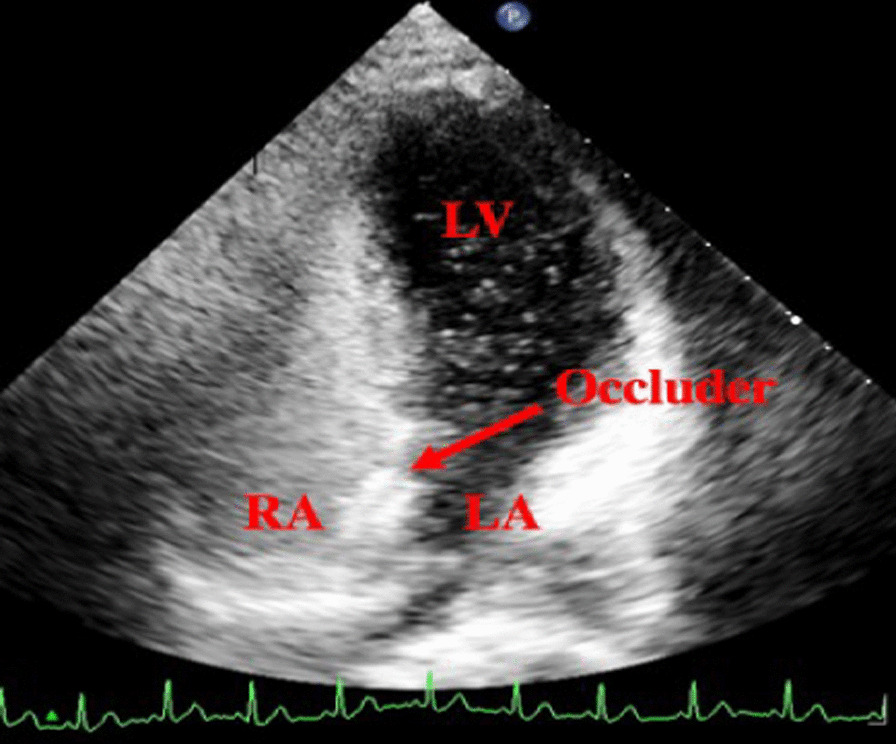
Positive transthoracic UFT result after PFO occlusion. There are more than 25 microbubbles in the LA and LV. *LV* left ventricular, *RA* right atrial, *LA* left atrial, *PFO* patent foramen ovale, *UFT* ultrasound foaming test
